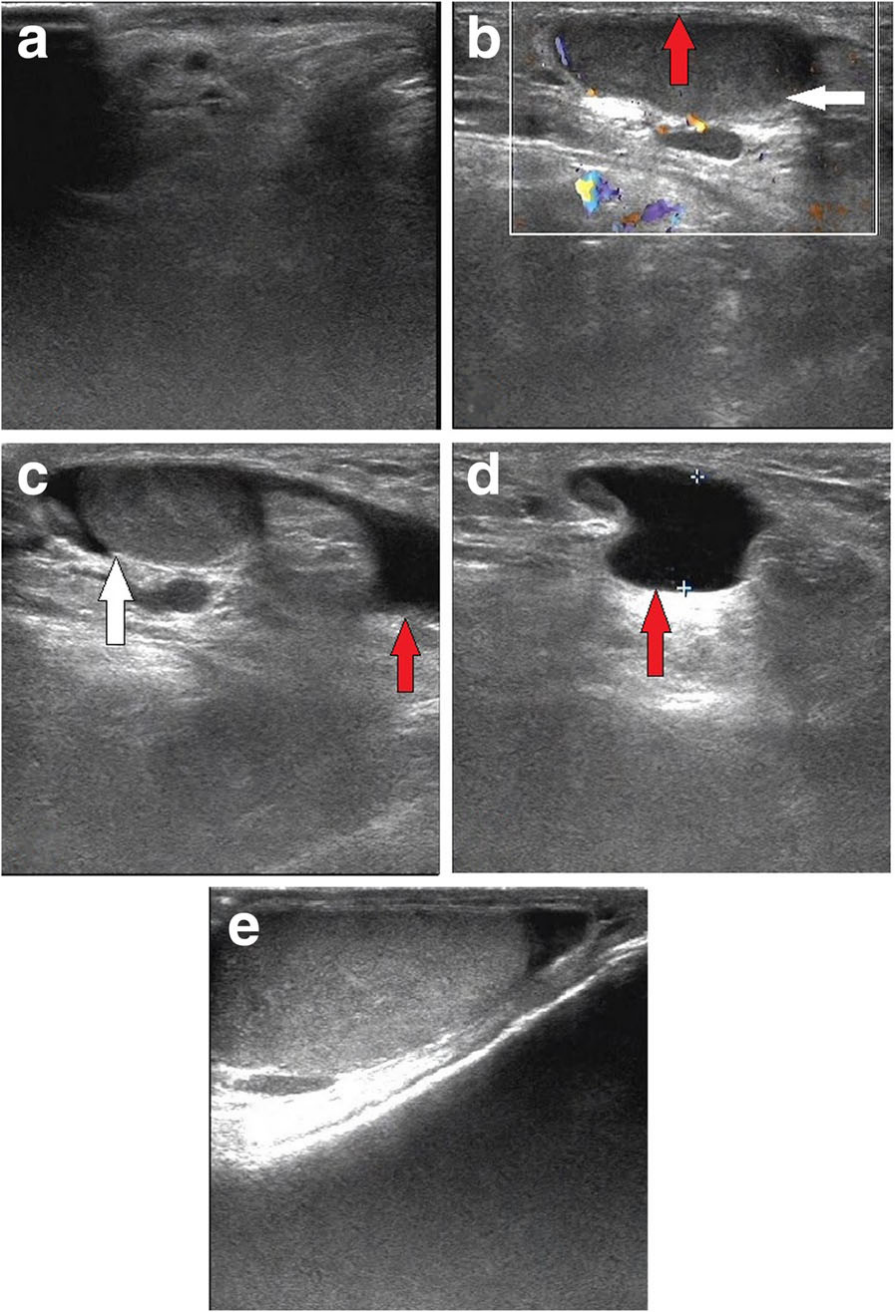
The ultrasonography of the testes. There was no testis in the right scrotum (a). There was an undescended testis in the right inguinal area, which was approximately 3.01 cm × 1.09 cm in size (b, white arrow). There was a thin layer of tissues with abnormal signal with dense echo in the peripheral part of the testis (red arrow). There was effusion (c, d, red arrow) adjacent to the testis (c, the white arrow). The left testis was seen in the left scrotum and no mass was found (e). The left testis was about 3.0 cm and relative small
